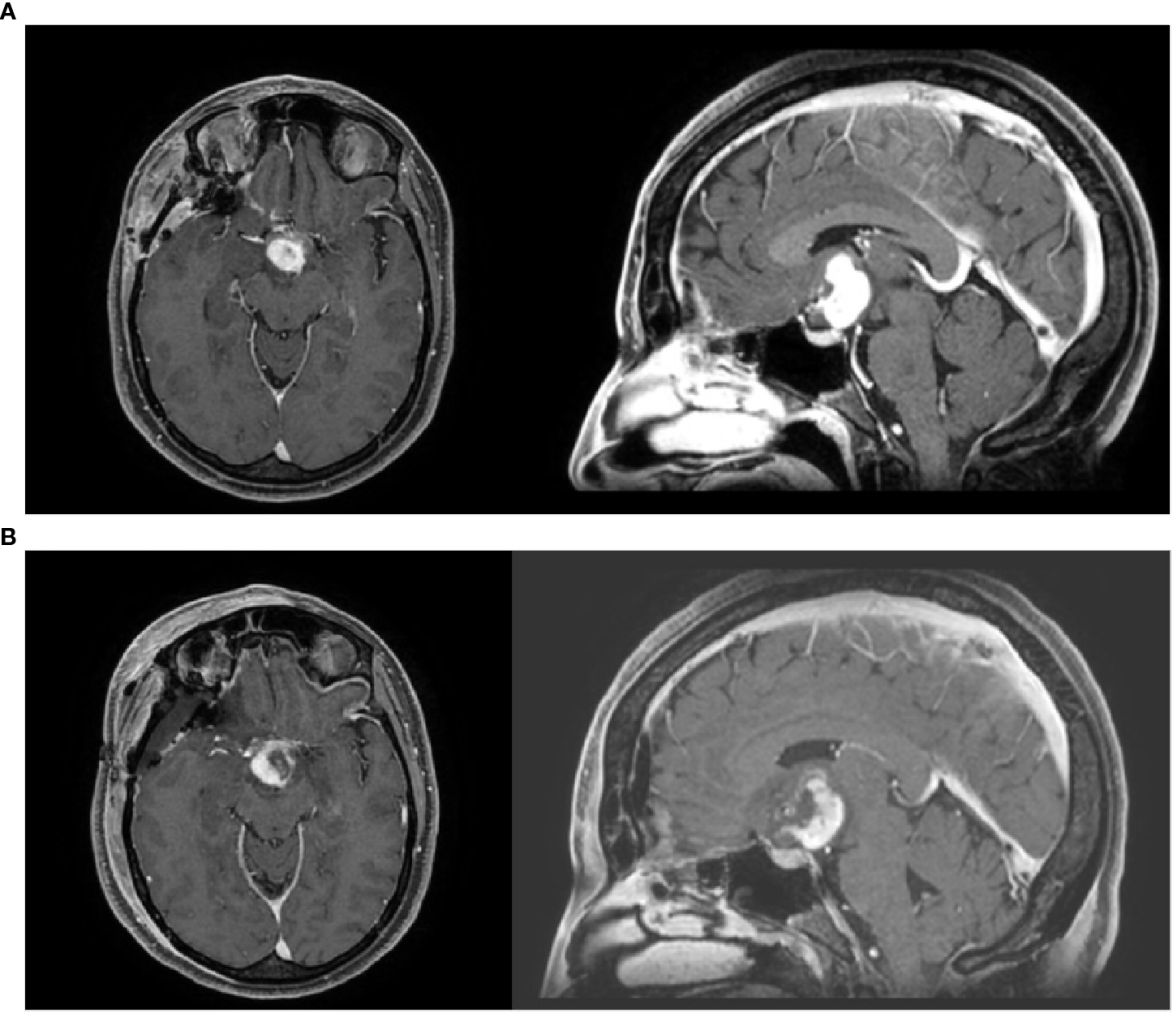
(A, B) Pre-operative and post-operative MRI imaging.
